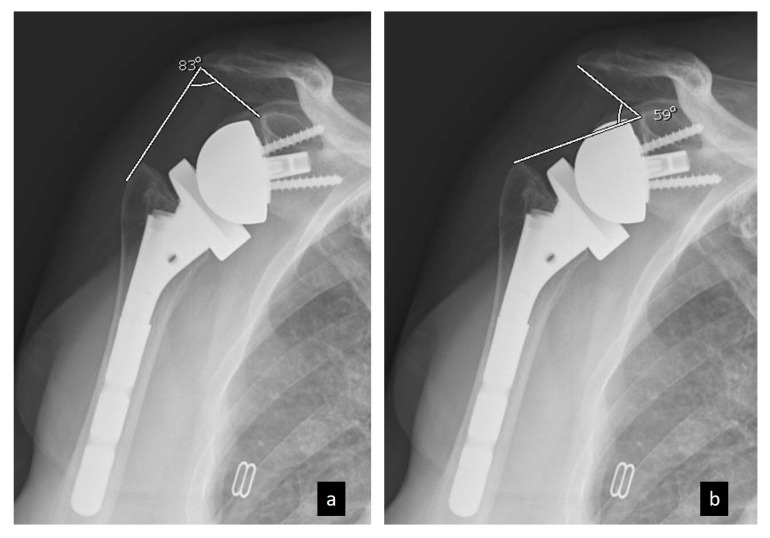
Radiological measurements of (a) lateralization shoulder angle (LSA) and (b) distalization shoulder angle (DSA) on a true anteroposterior shoulder radiograph.
